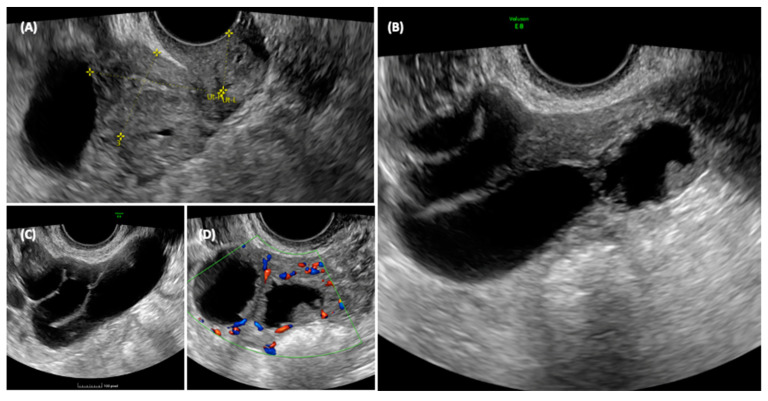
Ultrasonographic appearance of the pelvis. In the transvaginal scan, the anteverted uterus is visible in the longitudinal section (A), with a size compatible with the patient’s age. At the fundus of the uterus, a cystic formation is visible, closely adhering to the body of the uterus, and posterior to the uterus, the solid component of the formation can be seen. The detail of the cyst formation is visible in images (B,C). A unilocular cyst formation with incomplete septa is observed. In image (B) it appears that there is tissue protruding into the lumen of the cyst, mimicking the characteristic cog-wheel sign typical of inflammatory pathology, but when images are obtained in different planes (C,D) it is clear that this is in fact solid tissue, probably of a neoplastic nature, intensely vascularized.
